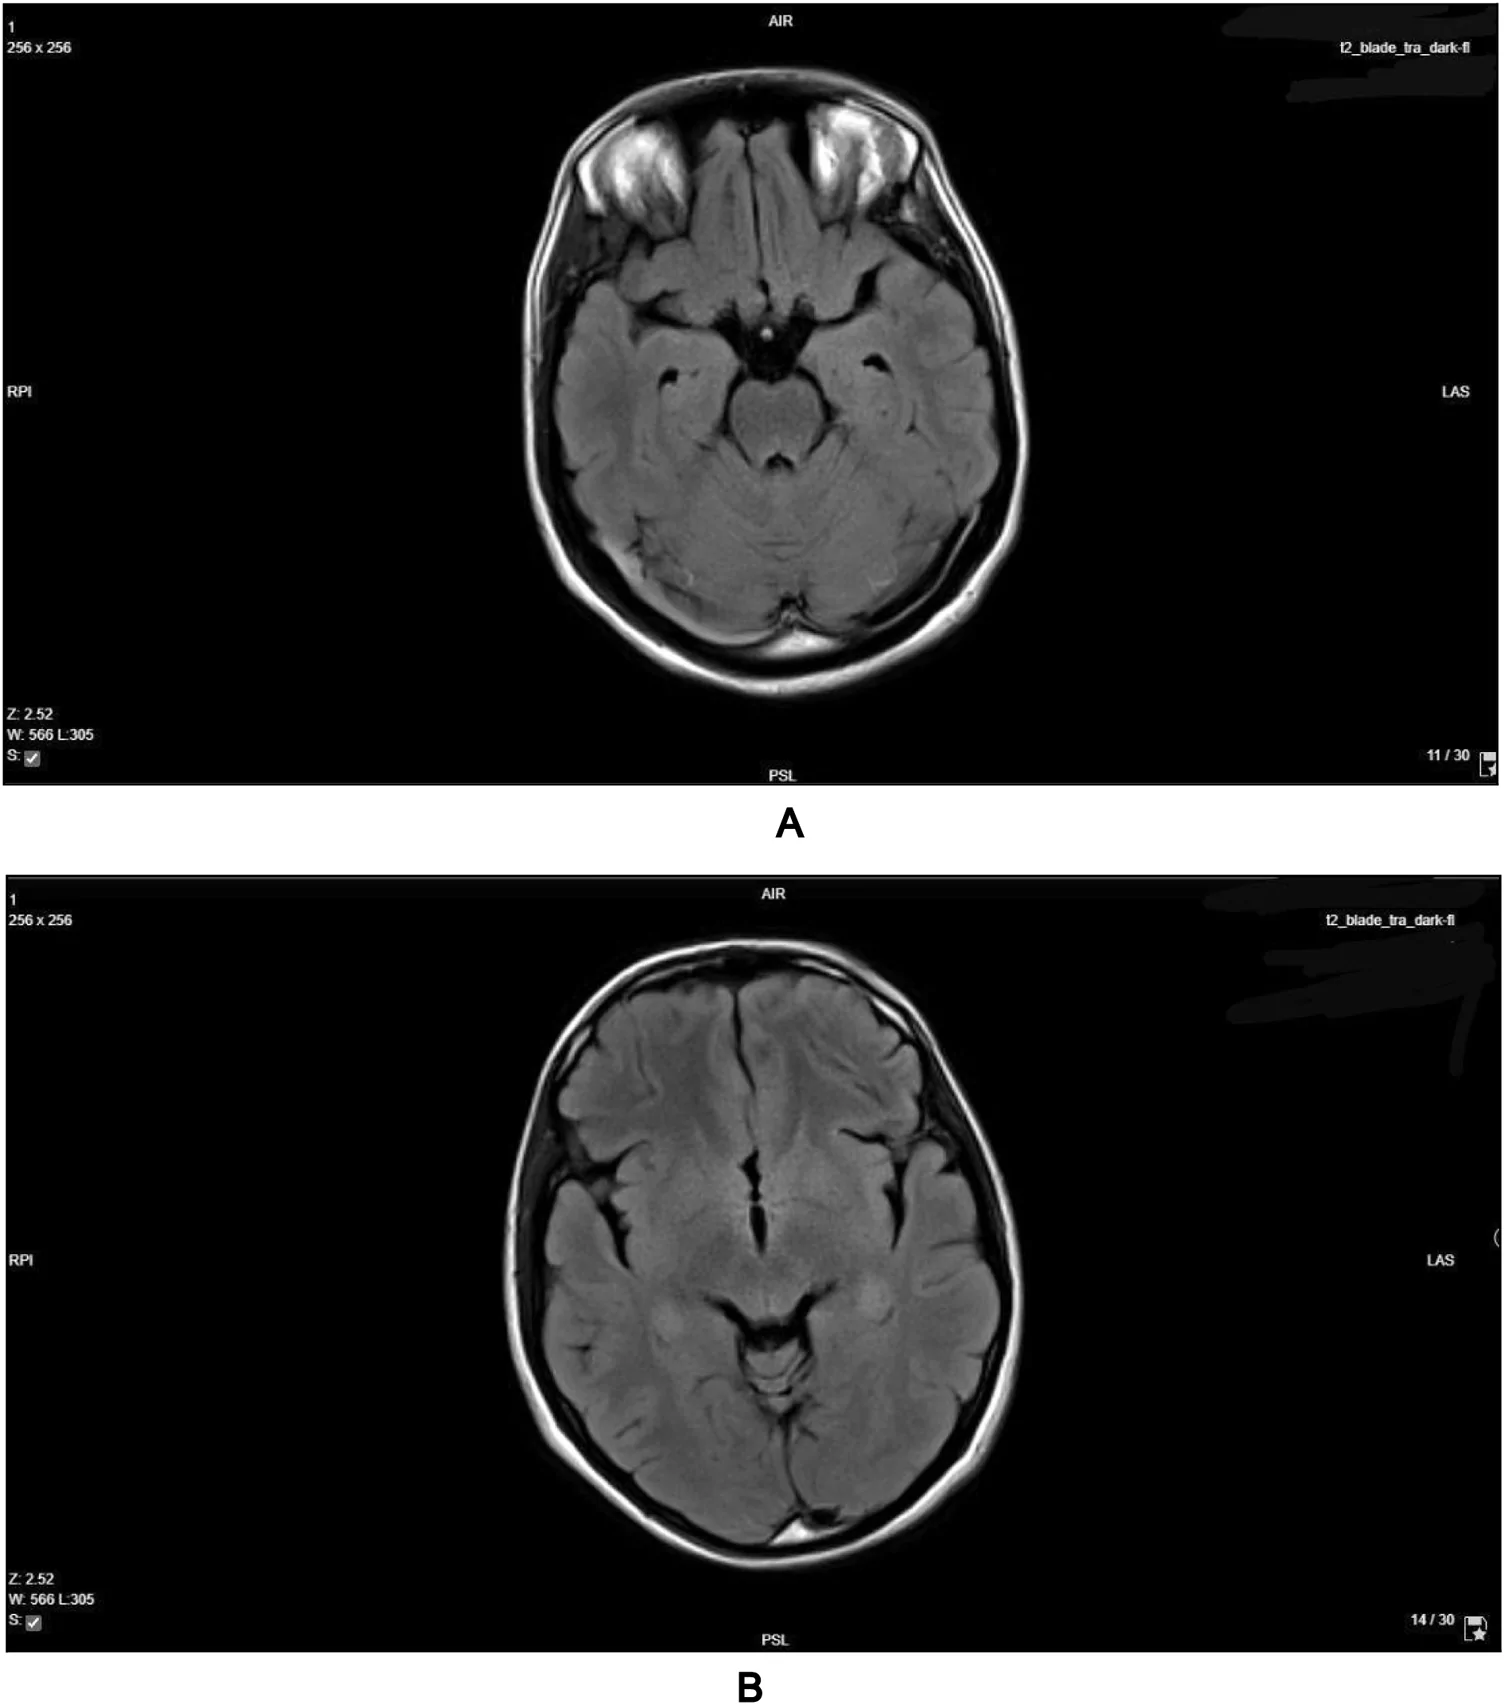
(A,B). Brain MRI obtained after TPE sessions (axial FLAIR) demonstrating no Acute Bilirubin Encephalopathy-pattern or basal ganglia involvement. (A,B) Axial FLAIR images at different levels demonstrate no definite abnormal signal intensity within the basal ganglia or other findings suggestive of bilirubin-induced neurologic dysfunction.
